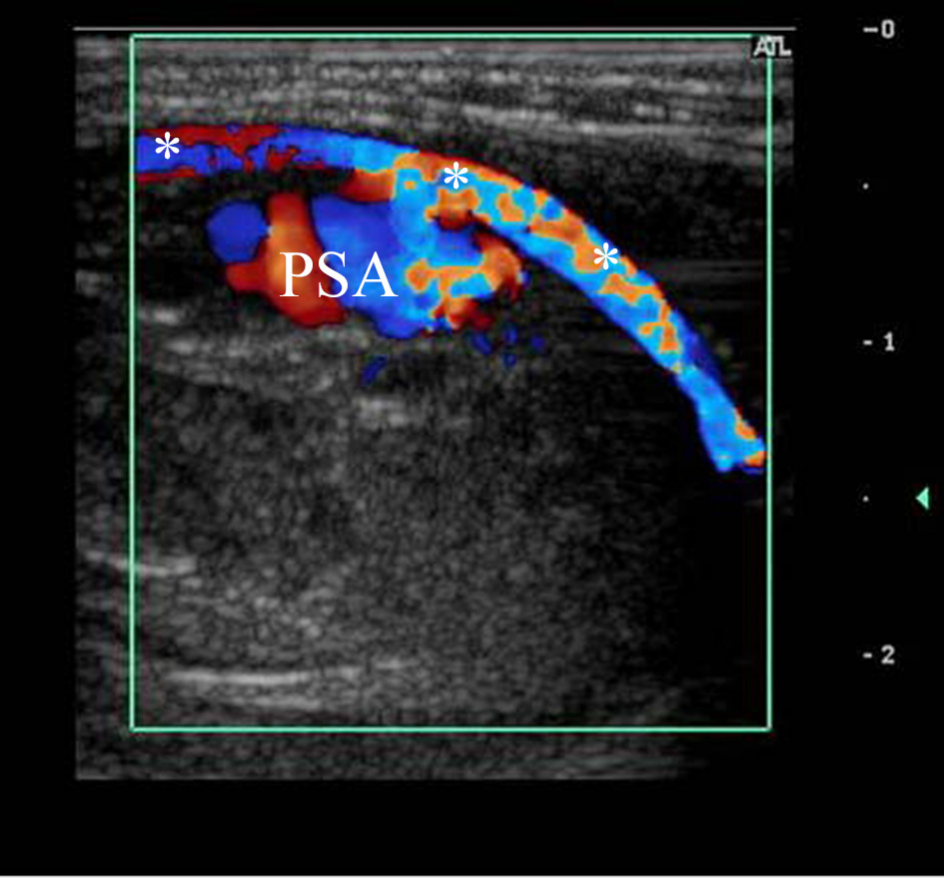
Colour Doppler study in a longitudinal plane after the second thrombin injection. It shows that the PSA reopened again with normal perfusion of the arm, normal flow through the brachial artery (*) and without loss of function. PSA, pseudoaneurysm.
